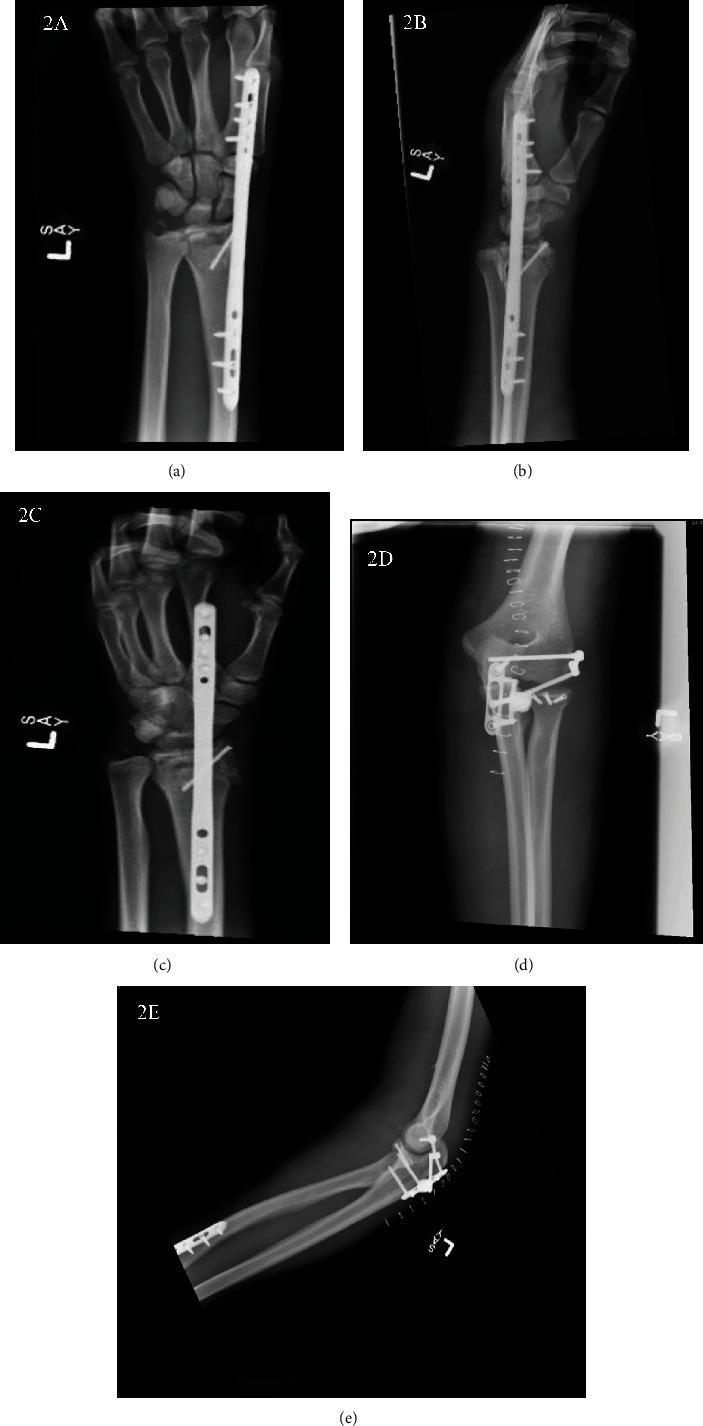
Postoperative radiographs of the plate fixation in the left wrist PA (a), lateral rotation (b), and external rotation (c) along with postoperative AP (d) and lateral radiographs (e) of the left elbow showing IJS placement.
